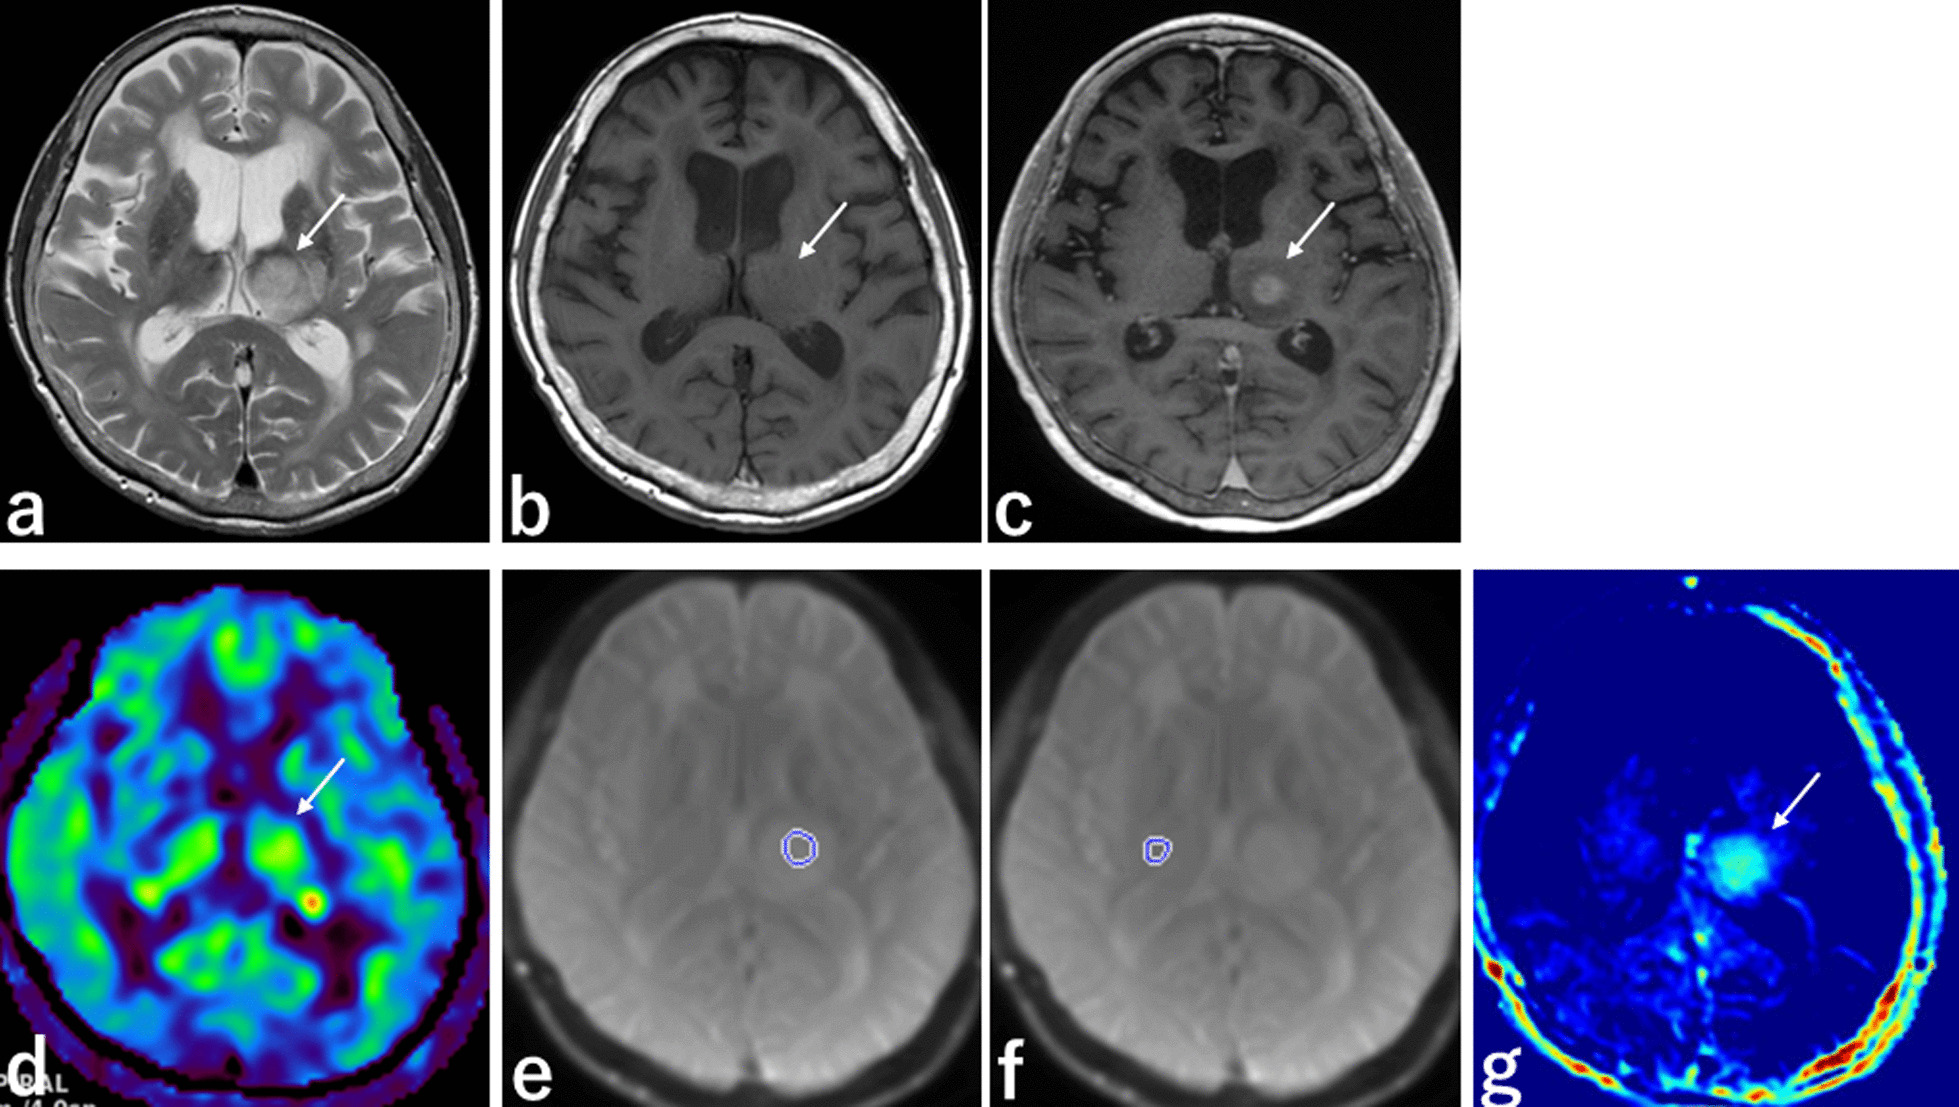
MRI from a 75-year-old woman with PCNSL. a T2-weighted sequence, axial section, shows well-defined PCNSL with high SI in the left thalamus (white arrow). b T1-weighted sequence, axial section, shows well-defined PCNSL with isointensity in the left thalamus (white arrow). c 3D contrast-enhanced T1-weighted sequence, axial section, shows enhancement in the center of PCNSL (white arrows). d 3D ASL sequence, axial section, shows isointensity in PCNSL. e APT scout image, axial section, shows the ROI placed in PCNSL. f APT scout image, axial section, shows the ROI placed in normal-appearing tissue contralateral to lesion. g APT imaging shows high SI in PCNSL (white arrow). *PCNSL* primary central nervous system lymphoma, *SI* signal intensity, *ASL* arterial spin labelling, *APT* amide proton transfer
